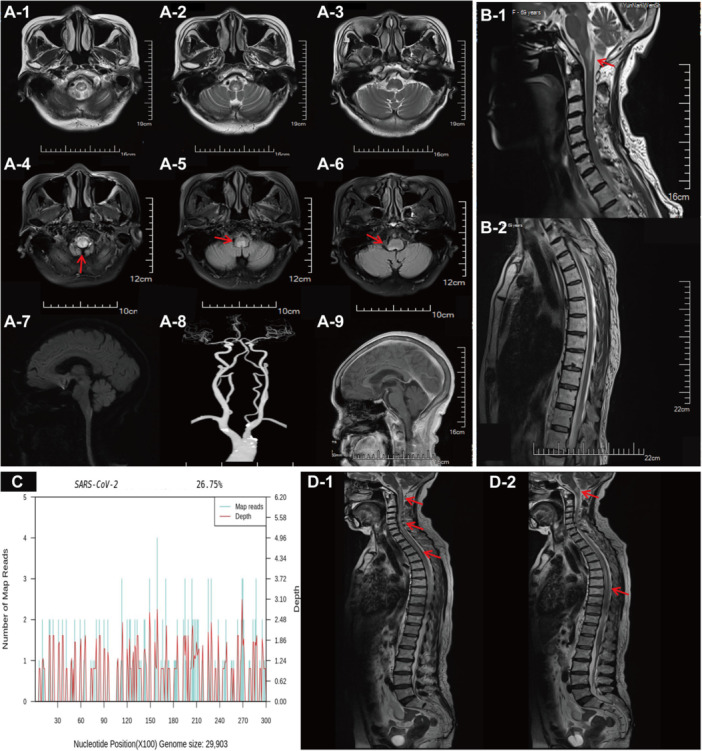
Patient 2's baseline craniospinal MRI on April 20, 2024, cerebrospinal fluid mNGS test results on May 2, 2024, and recurrent craniospinal MRI on October 17, 2024. (A1–6) Brain MRI shows T2/FLAIR hyperintensity involving the dorsal medulla at the level of the cervicomedullary junction (V‐sign, arrows). (A7–9) Brain DWI, head‐neck CTA, and contrast‐enhanced brain MRI show no abnormalities. (B1–2) Sagittal spinal MRI reveals isolated T2 hyperintensity posterior to the medulla‐spinal cord junction. (C) Cerebrospinal fluid mNGS on May 2, 2024, detected 148 SARS‐CoV‐2 RNA gene sequences with a relative abundance of 26.75%. (D1–2) Six months after discharge, the patient developed lower limb weakness and sphincter dysfunction, with follow‐up MRI showing longitudinally extensive transverse myelitis (LETM) extending from the medulla to the thoracic spinal cord.
